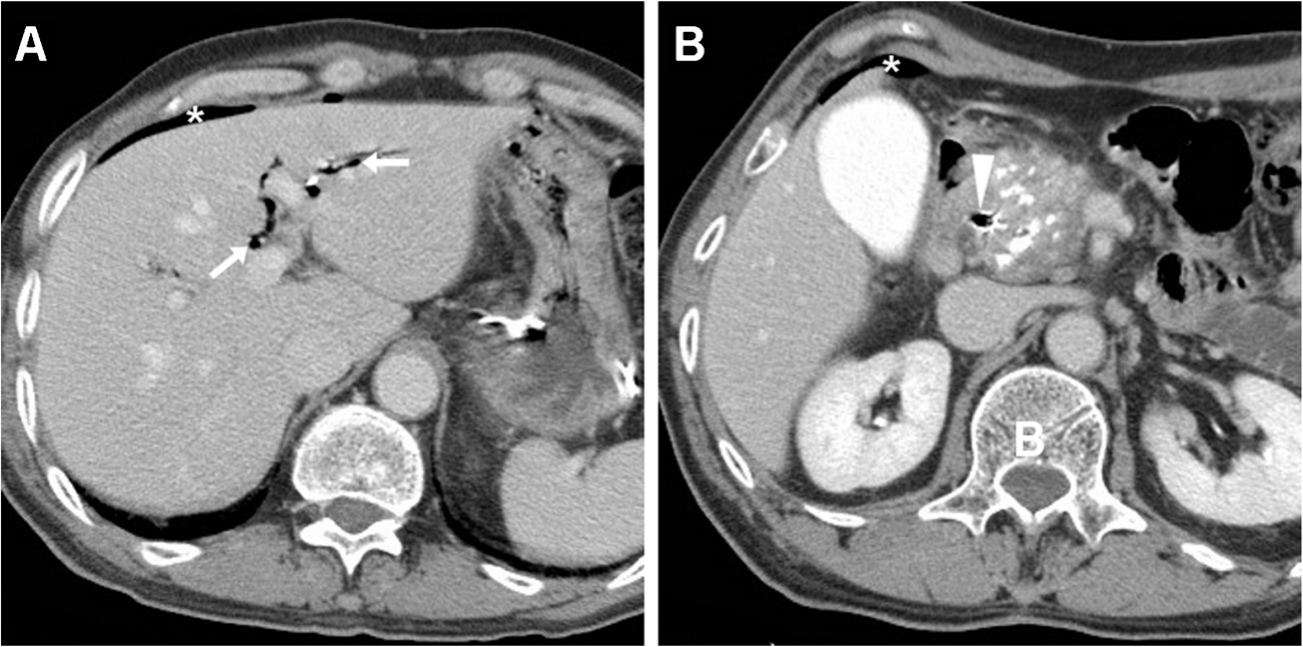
In a 60-year-old male with severe chronic calcific pancreatitis, after ERCP positioning of the metallic pancreatic stent (*arrowhead* in a) contrast-enhanced CT showed minimal intraperitoneal air (*), which was treated conservatively with success. Note pneumobilia (*arrows*) and retained CM in the gallbladder
